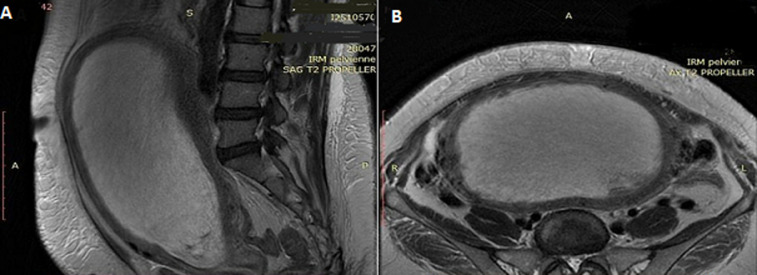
sagittal and axial magnetic resonance imaging sections showing an enlarged uterus with a microcystic and hemorrhagic intracavitary process
